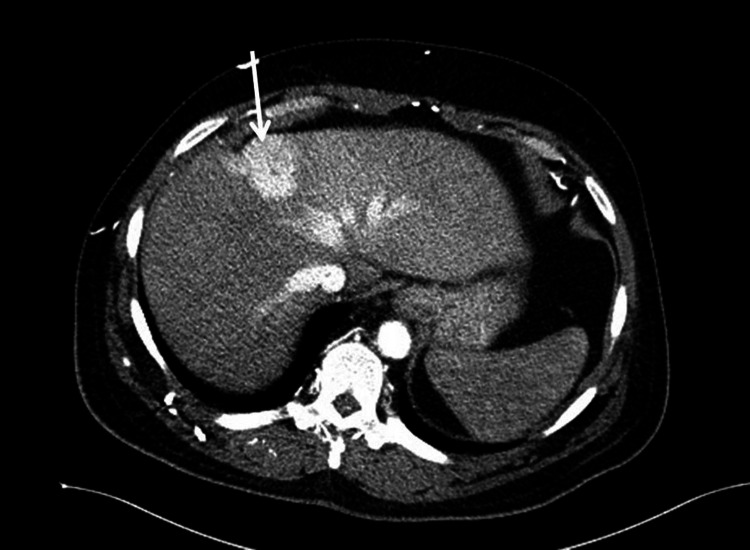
Contrast-enhanced CT of the abdomen demonstrating intense arterial hyperenhancement of the hepatic quadrate lobe
